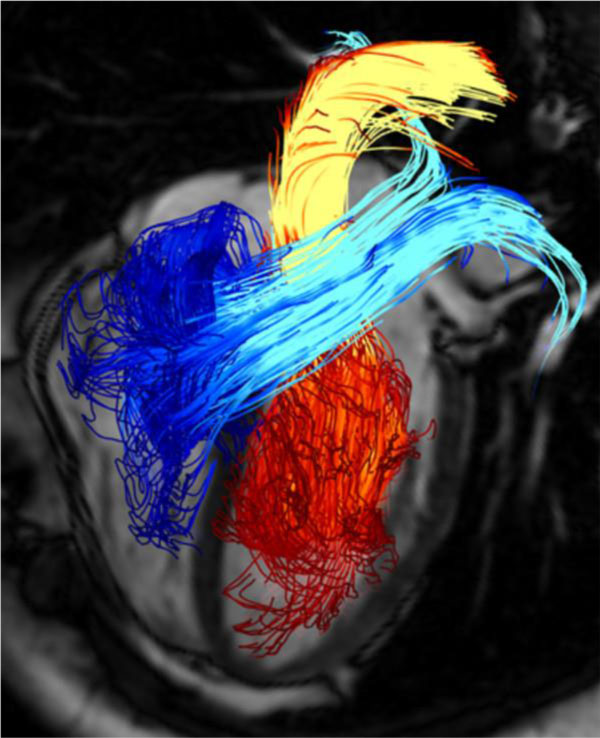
Pathline visualization of cardiac blood flow using 4D phase contrast MRI. Pathlines are originated from planes at the mitral valve (red-yellow) and the tricuspid valve (blue-turquoise) at early diastolic ventricular inflow. A separately acquired balanced steady-state free precession three-chamber image was superimposed for providing anatomical orientation [67].
